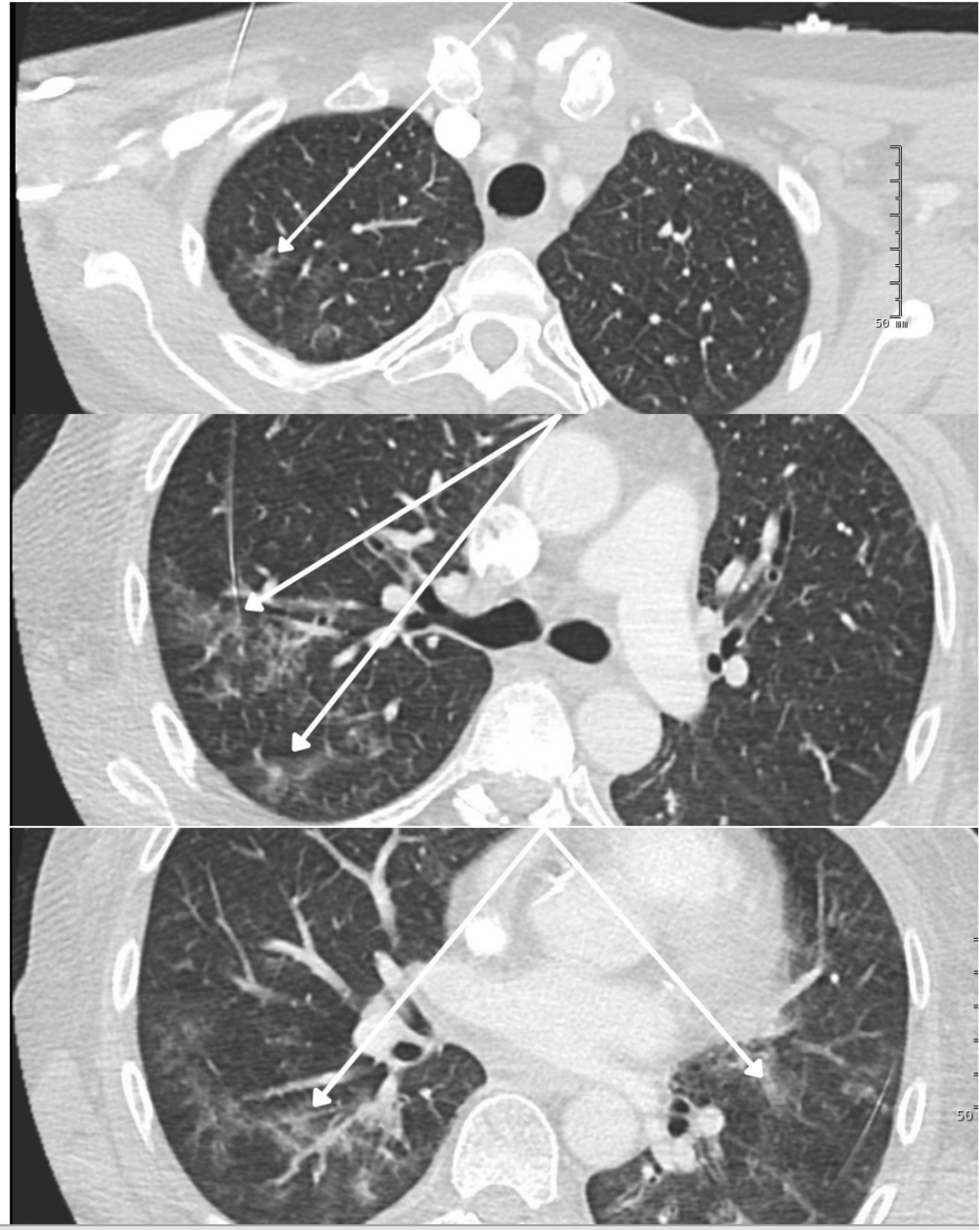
Selected Cuts of the CT Angiogram of the Chest Multifocal, bilateral ground glass opacities are demonstrated, more prominent on the right than the left, which were noted in all five lobes of the lung.
